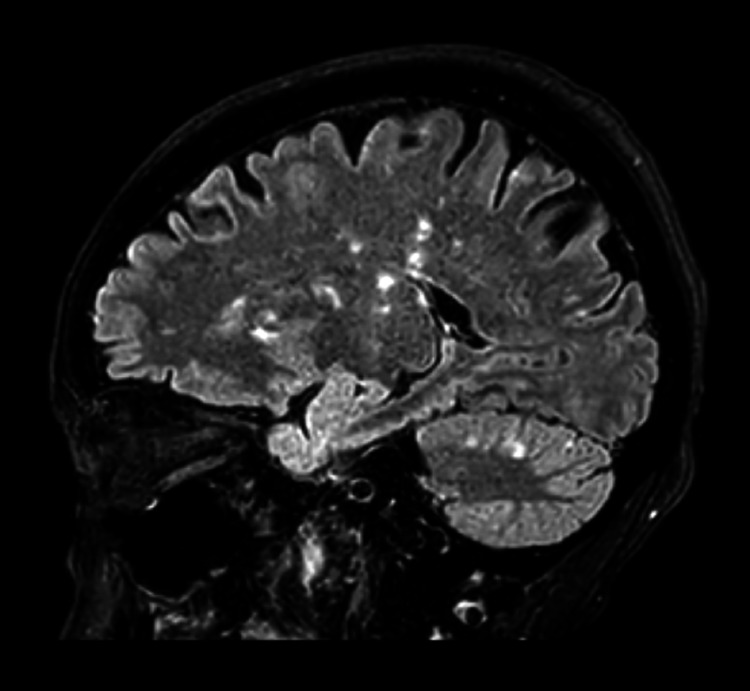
MRI brain with multiple infarctions in both the cerebrum and cerebellum MRI: magnetic resonance imaging
